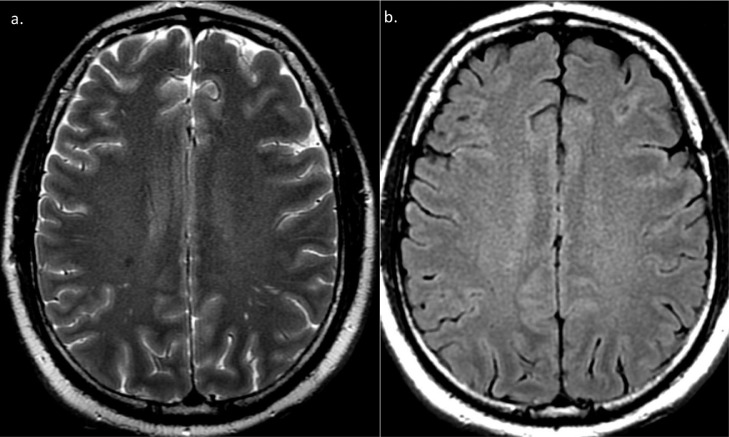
T2 (a) and FLAIR (b) MRI sequences at the level of centrum semiovale showing normal appearance on initial MRI.
